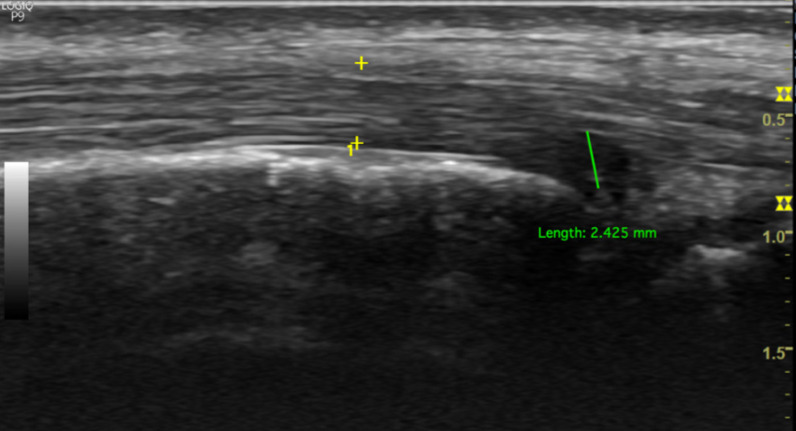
Longitudinal sonographic of posterior tibialis tendon with partial tear.
